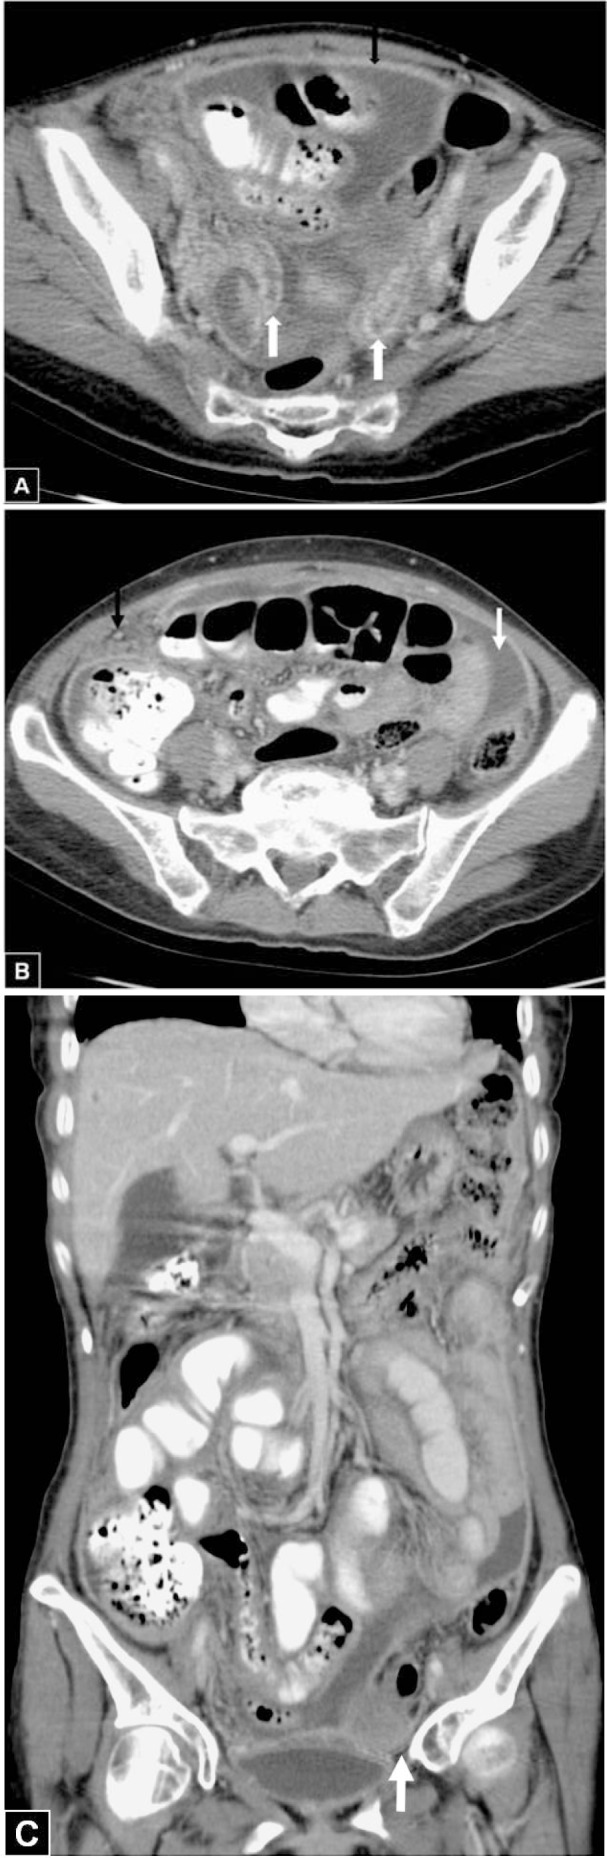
56-year-old woman had suffered from abdominal fullness for three months. (A) Axial contrast-enhanced abdominopelvic CT scan shows a uniform well-enhanced peritoneum (black arrow), and bilateral dilated convoluted fallopian tubes with intense mucosal enhancement (white arrow) representing bilateral salpingitis; these findings combined with dirty fat strandings identify infection. (B) Axial contrast-enhanced CT scan shows the nodules of the omentum (black arrow) and loculated ascites (white arrow). (C) Coronal contrast-enhanced CT scan demonstrates the disproportionate left ovarian mass (arrow) with loculated ascites, in contrast to the usual findings of ovarian cancer.
